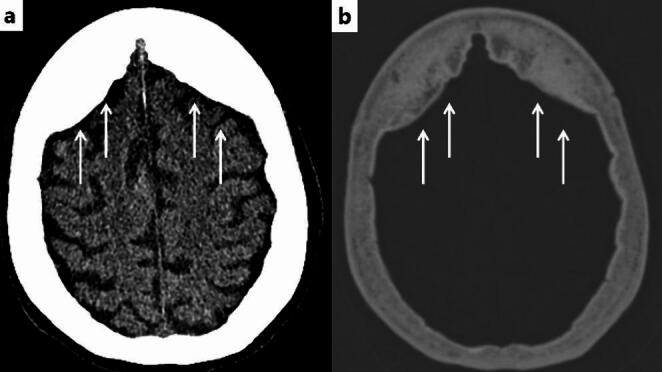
Hyperostosis frontalis interna (HFI). a CT, b CT (bone window). Non-contrast CT in the bone window demonstrated symmetric, smooth thickening of the inner table of the frontal bone with preservation of the outer table and diploic space. This benign finding is frequently associated with metabolic disorders and should not be mistaken for Paget’s disease or tumor-related hyperostosis. Abbreviation: CT, computed tomography; HFI, Hyperostosis frontalis interna
